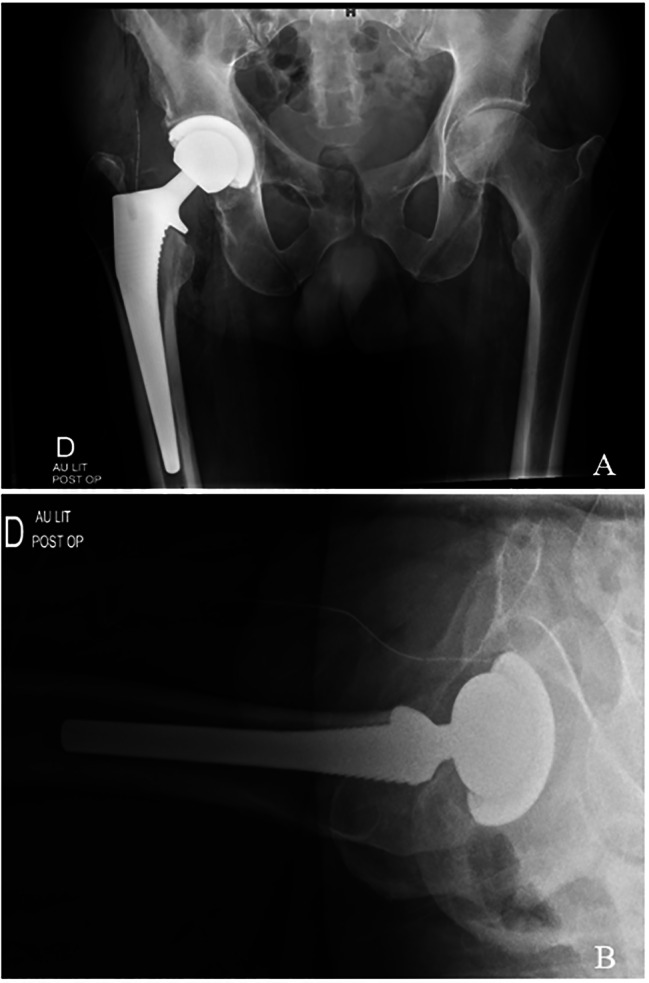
Postoperative X-Ray with Anteroposterior view (A) and Lateral view (B)
